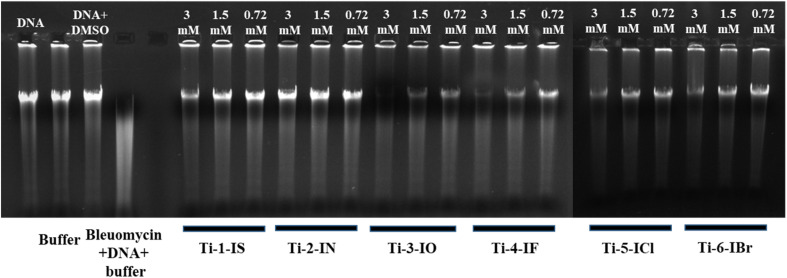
Agarose gel electrophoresis of CT-DNA with Ti(iv) complexes (1) CT-DNA in PBS buffer, (2) CT-DNA in PBS + DMSO, (3) PBS, (4) CT-DNA in PBS + bleomycin, (5) lanes 5–18 CT-DNA + (3, 1.5 and 0.75 mM) Ti-1-IS to Ti-6-IBr.
